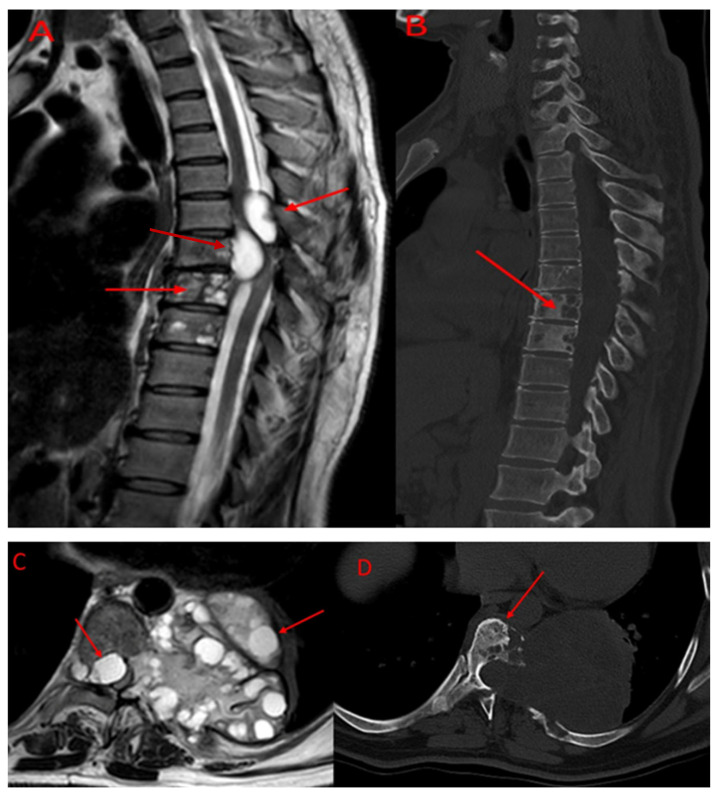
Preoperative imaging of thoracic spinal hydatid cyst. (A): Sagittal T2-weighted MRI demonstrating extensive thoracic vertebral involvement by multiloculated hydatid cysts with high signal intensity. Arrows indicate cysts extending into the spinal canal, causing cord compression, as well as multivesicular involvement of the vertebral body. (B): Corresponding sagittal CT scan of the same patient. The arrow indicates cortical bone destruction and vertebral involvement. (C): Axial section of the MRI shown in Figure 3A. The arrows indicate a cyst compressing the spinal cord and paravertebral cystic involvement. (D): Axial CT image corresponding to Figure 3B, showing cortical bone destruction and vertebral involvement. Abbreviations: MRI, magnetic resonance imaging; CT, computed tomography.
